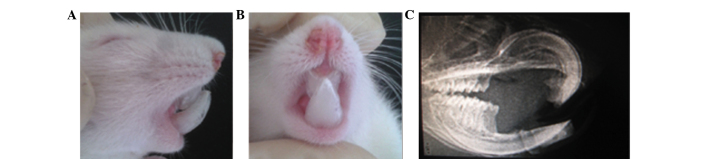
(A) Lateral and (B) frontal views and (C) a radiograph following fitting of a functional applicance showed vertical and anterior displacement of the mandible.
